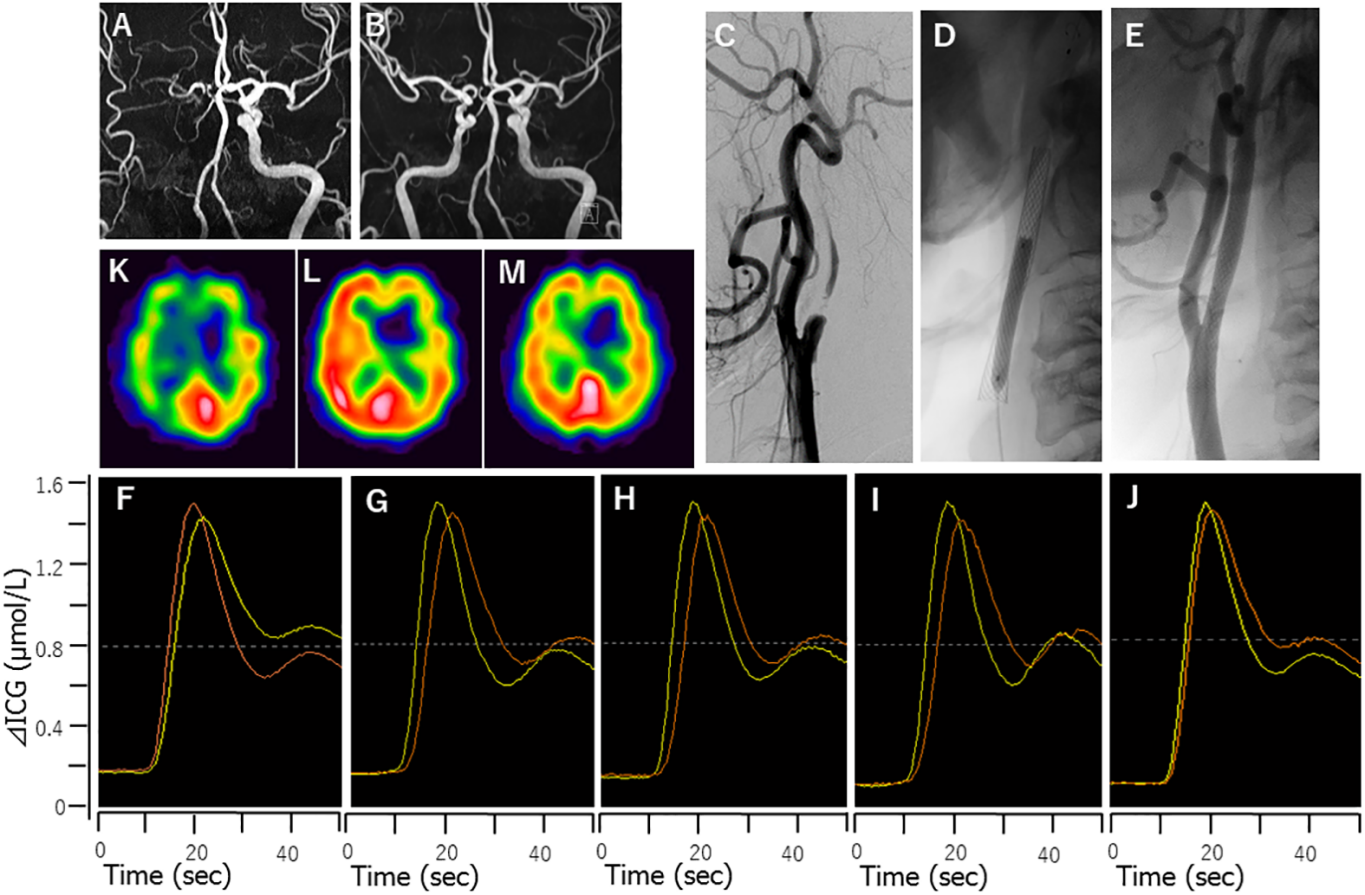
Case 2 (HPS case): A 70-year-old male presented with symptomatic right internal carotid artery stenosis. Magnetic resonance angiography showed reduction in the right internal carotid artery signal before (A) and after CAS (B). He underwent right-side CAS with NIRS monitoring. Right common carotid angiography showed right internal carotid artery nearly occlusion (C). Balloon angioplasty for post-dilatation was performed after stent placement with filter protection (D). Right common carotid angiography after CAS confirmed stent patency (E). MRI showed no additional ischemic lesions, and hence, he was diagnosed with postoperative HPS. The patient’s systolic blood pressure was strictly controlled between 100–140 mmHg, and sedation was induced with dexmedetomidine. Seven days after CAS, he recovered from the symptoms and he was discharged without any neurological deficits. Time-intensity curve from ICG-NIRS monitoring before (F), just after (G), 6 hours after (H), 1 day after (I), and 7 days after CAS (J). A remarkable change in the time-intensity curve time just after CAS was observed; the difference disappeared 7 days after CAS. Yellow curve; affected side, Orange curve; unaffected side. Preoperative ^123^I-IMP SPECT in the resting state showed a decrease in rCBF in the right hemisphere (K). One day after CAS, hyperperfusion was seen in the ipsilateral hemisphere (L). One week after CAS, hyperperfusion in the ipsilateral hemisphere had normalized (M).
